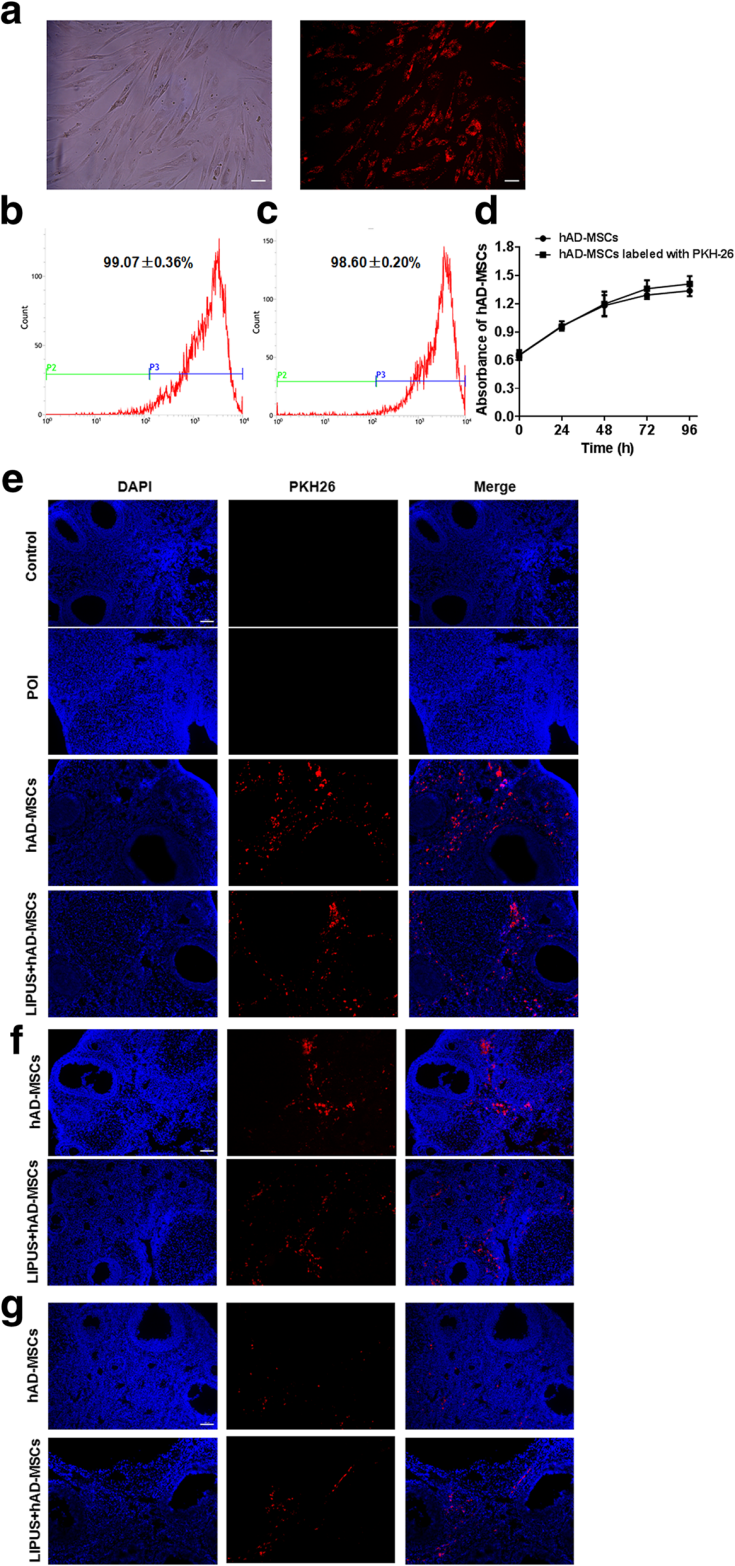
In vivo hAD-MSC tracking. a PKH26-labeled hAD-MSCs showed red fluorescence (100×). b,c The labeling rates of PKH26-labeled hAD-MSCs (b) and their subcultured cells (c) were detected by flow cytometry. d The growth curves of PKH26-labeled and unlabeled hAD-MSCs were measured by CCK-8 assay (*n* = 6). e–g Transplanted hAD-MSCs were observed at 24 h (e), 4 weeks (f), and 8 weeks (g) after cell transplantation in ovaries (100×). *Scale bars* = 100 μm. P* < 0.05, *P* < 0.01. *hAD-MSCs* human adipose-derived mesenchymal stem cells, *LIPUS* low-intensity pulsed ultrasound, *POI* primary ovarian insufficiency
